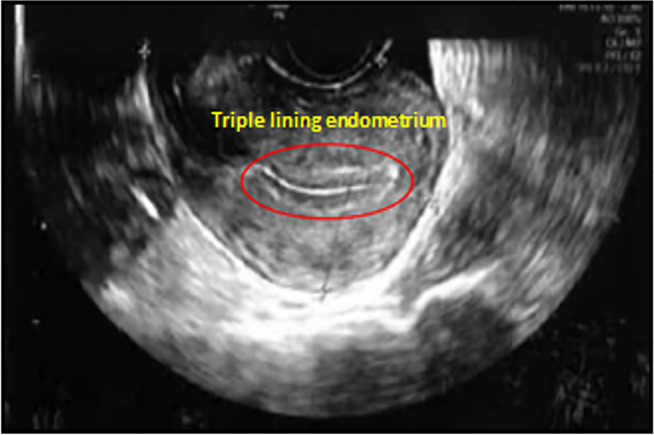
Transvaginal ultrasound examination showing endometrial lining after
